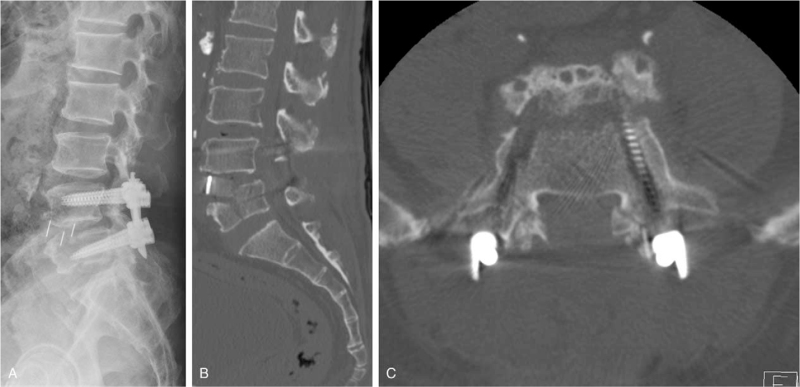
Preoperative imaging for revision operation following sudden back pain and radiating pain in the left leg. (A–C) Radiography and computed tomography show a split coronal fracture of the L5 body and an anterior cage displacement.
